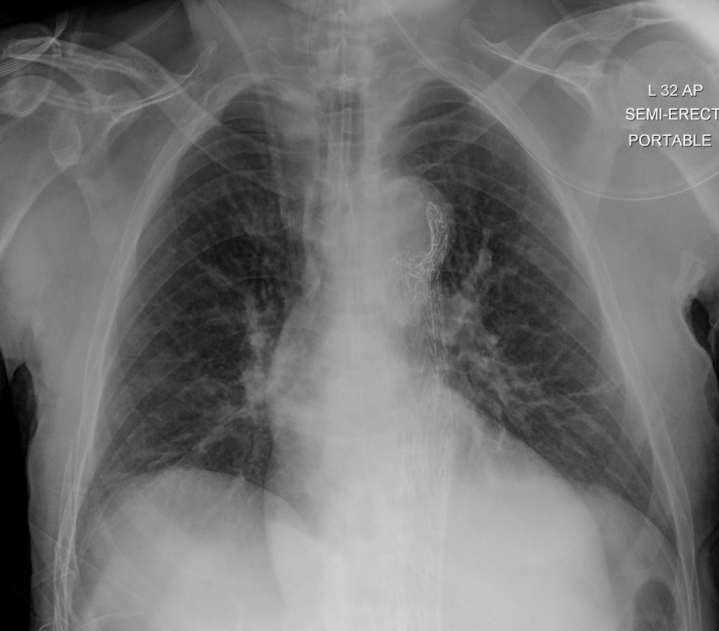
Postoperative day 1 chest radiograph demonstrating collapse of proximal segment of thoracic endovascular aortic repair stent.
